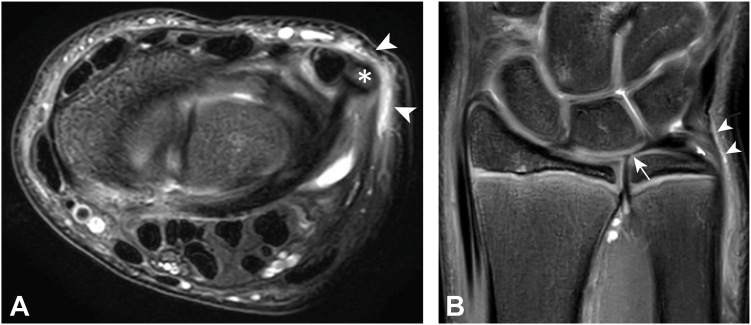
T2-weighted fat saturated MRI of the same asymptomatic gymnast showing subcutaneous fat infiltration (arrow heads) surrounding the ulnar styloid process (asterisks) suggesting a sort of ulnar wrist impingement. A Axial T2-weighted fat saturated MRI. B Coronal T2-weighted fat saturated MRI. Note the vertical linear increased signal intensity within the articular disc (arrow), which was observed in nongymnasts as well as asymptomatic gymnasts.
